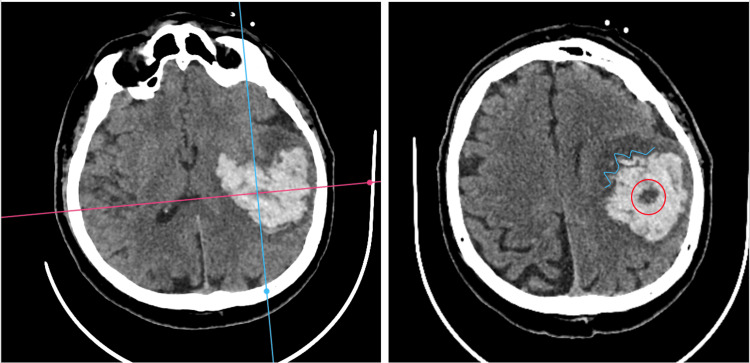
Cranial CT scan obtained at the emergency department Left: The head CT scan shows a 70 ml large left capuso-lenticular hematoma; Right: The head CT scan shows a black hole sign (red circle) and margin irregularities (light-blue line). Signs of active bleeding are noted.
